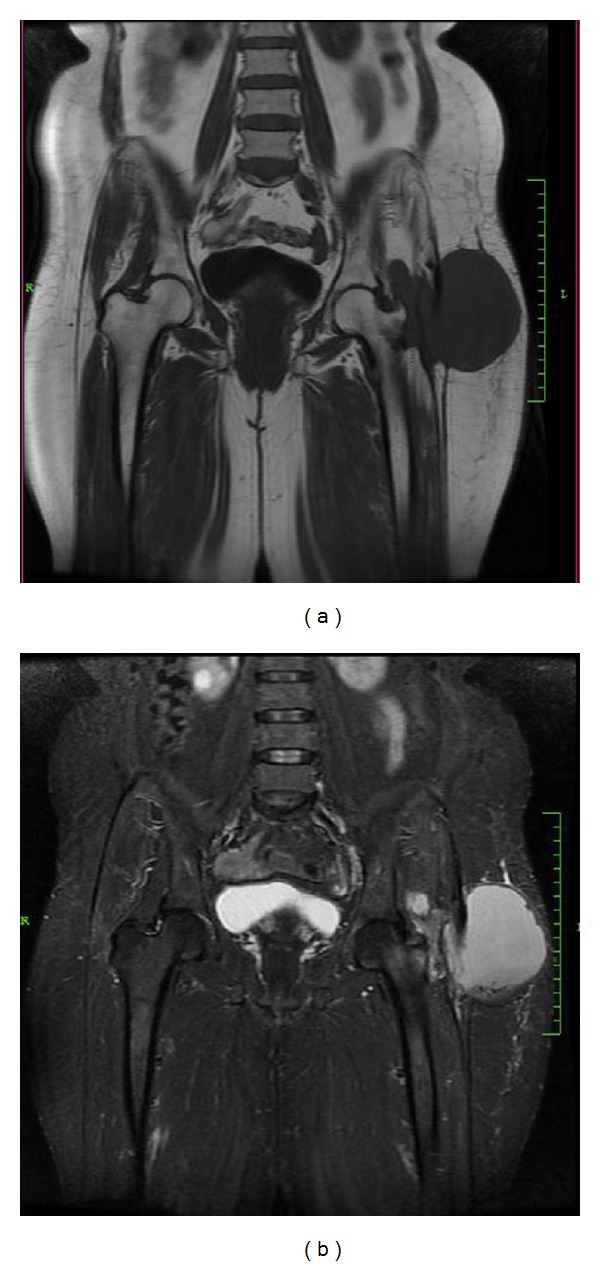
Coronal T1- and T2-weighted MRI image of the pelvis showing focal areas of altered marrow signal intensity changes appearing hypointense on T1-weighted image and hyperintense on T2-weighted image, suggestive of infective lesion. A large localized fluid collection is seen in the subcutaneous tissues of the lateral aspect of the left thigh.
